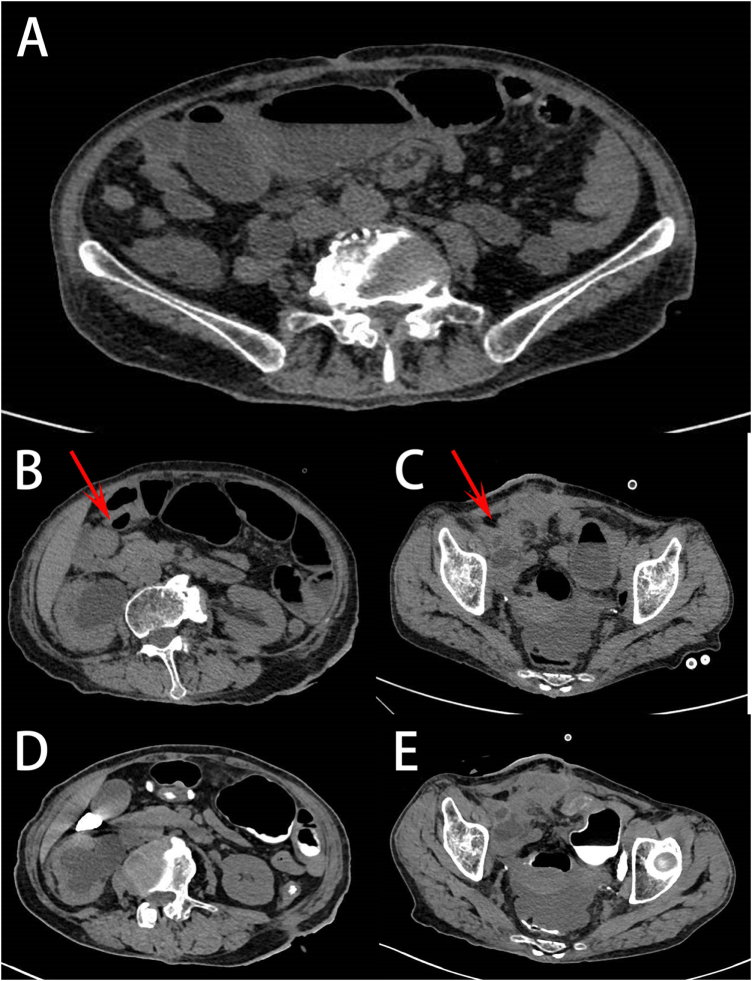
Follow-up CT imaging upon presentation with intestinal obstruction. (A) Emergency CT scan (axial view) reveals air-fluid levels (arrow) within dilated loops of the small intestine, corresponding to mechanical obstruction. (B and C) CT (axial and coronal views, respectively) scan at admission demonstrates the presence of soft tissue density mass (red arrow) in the right lower abdominal wall adjacent to the dilated intestine and the persistence of marked dilation of the right renal pelvis and ureter. (D and E) Contrast-enhanced CT images in axial and coronal planes clarify the obstructive mass and its relationship to surrounding structures.
